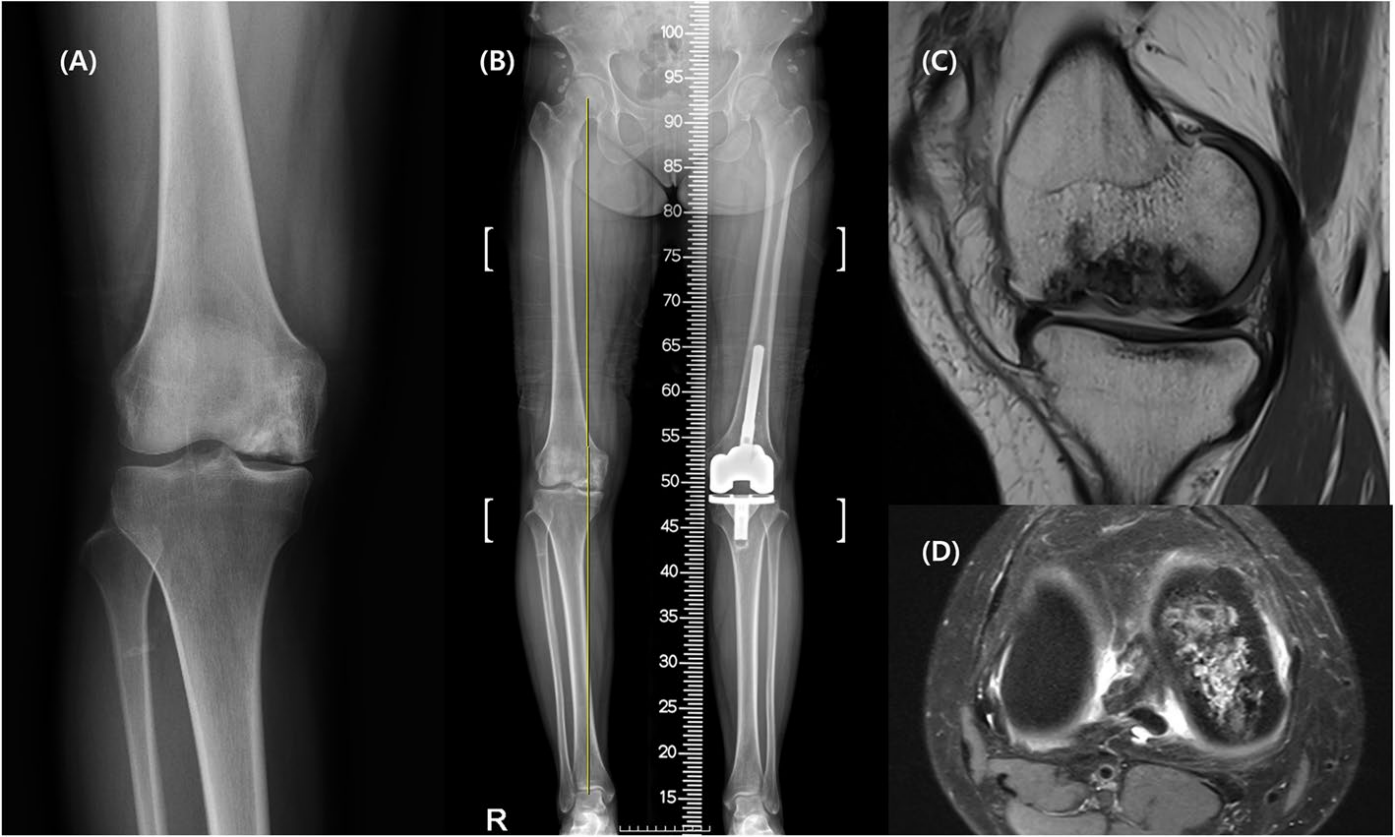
Preoperative imaging studies. A Anteroposterior radiograph of right knee. B Standing scanogram of whole lower limb demonstrating 8-degree varus alignment in the hip-knee-ankle axis. C Sagittal and (D) Axial magnetic resonance imaging of right knee showing large-sized collapsed osteonecrosis of the medial femoral condyle
